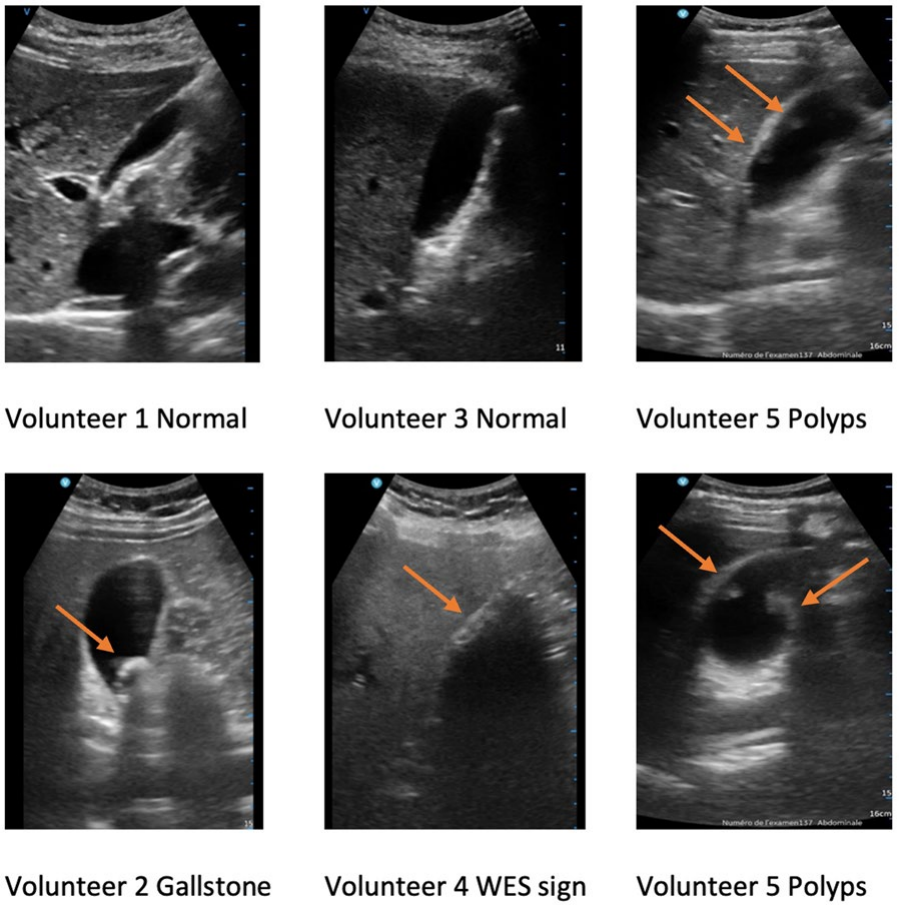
Gallbladder ultrasound images from the evaluation day. WES: wall-echo-shadow
